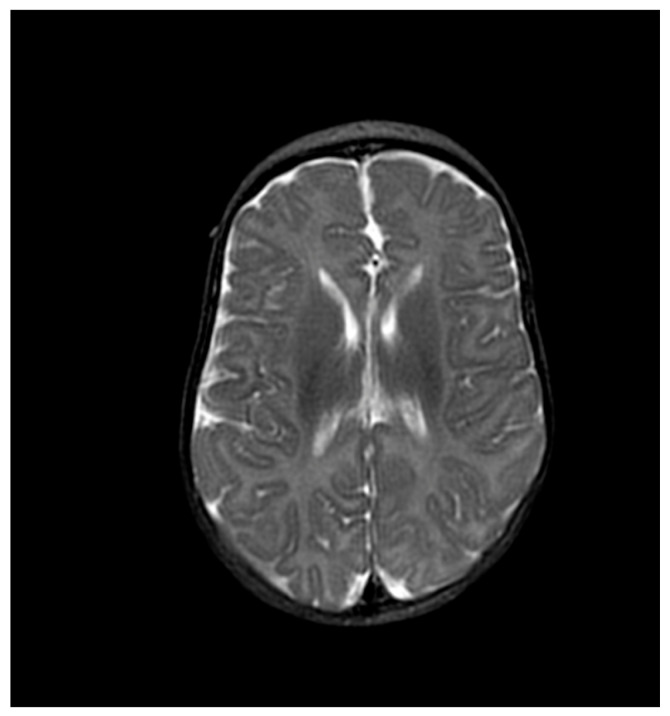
Brain magnetic resonance imaging (MRI) showing no pathological findings.
